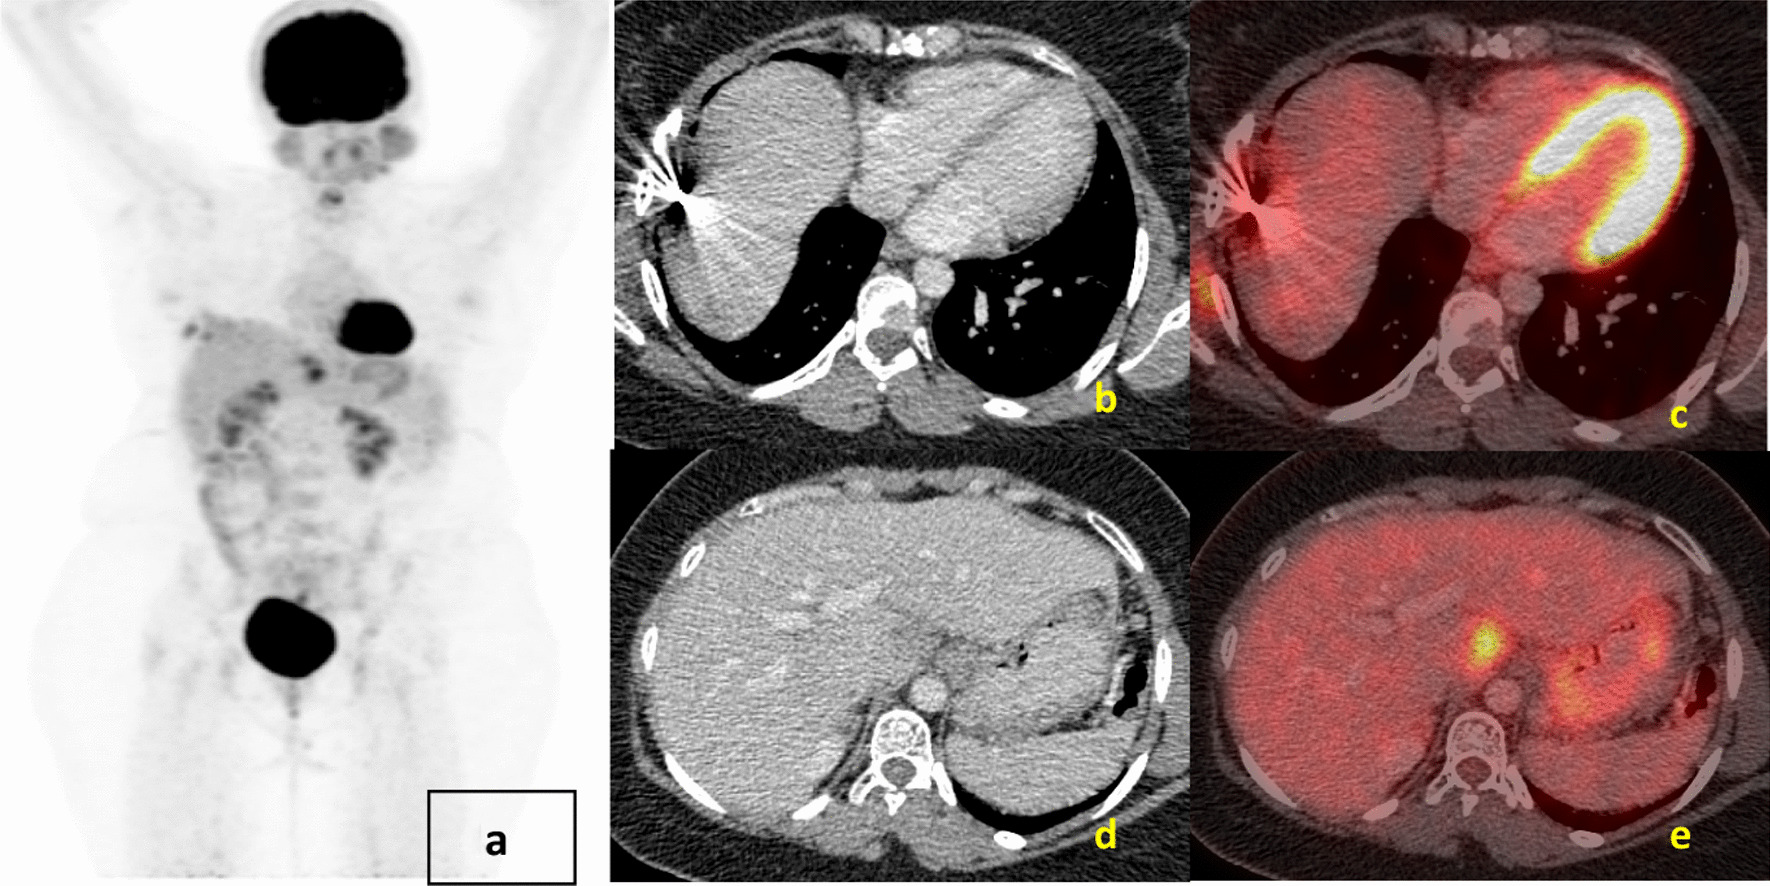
a Maximum-intensity projection image of fluorodeoxyglucose positron emission tomography - computerized tomography showing increased tracer uptake in the right upper abdominal region corresponding to artifactual uptake (c) in site of previously seen metastasis of liver (b). d Axial CT abdomen region showing hypodense lesion in caudate lobe of liver showing uptake on fused fluorodeoxyglucose positron emission tomography - computerized tomography image (e)
